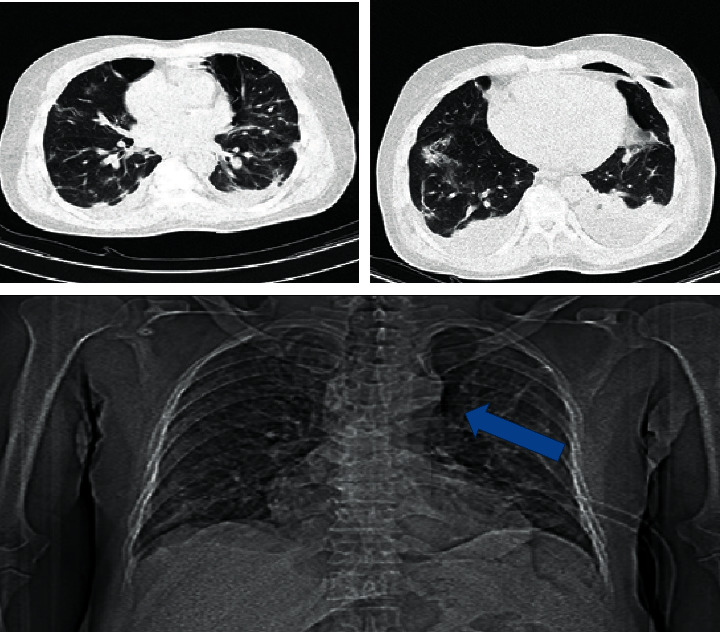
Chest CT showing spontaneous pneumomediastinum (arrow), emphysema, bilateral pleural effusion, and ground-glass opacities of the lung, which decreased after treatments.
